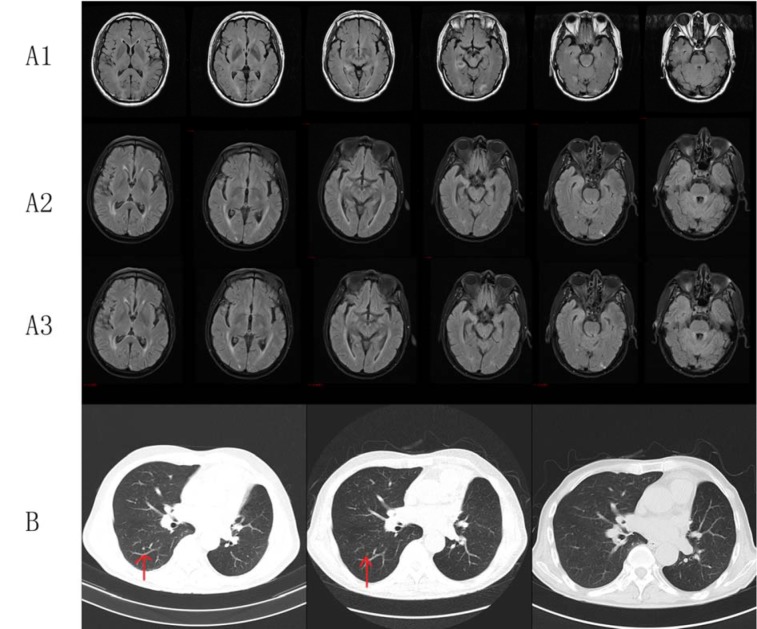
Clinical images of Case 5. (A1) Brain MRI showed multiple nodules in the brain, and metastatic tumor was considered; (A2) Brain MRI showed more ischemic foci and lacunar infarction in the bilateral frontal and parietal lobes and demyelination after 3 months of EGFR-TKI therapy; (A3) Brain MRI showed shrinking metastatic tumor in the brain, more and more ischemic foci and lacunar infarction in the bilateral frontal and parietal lobes, and demyelination after 6 months of EGFR-TKI therapy; (B) Chest CT with contrast of patient 5 showed the gradual disappearance of pulmonary nodules after EGFR-TKI treatment.
